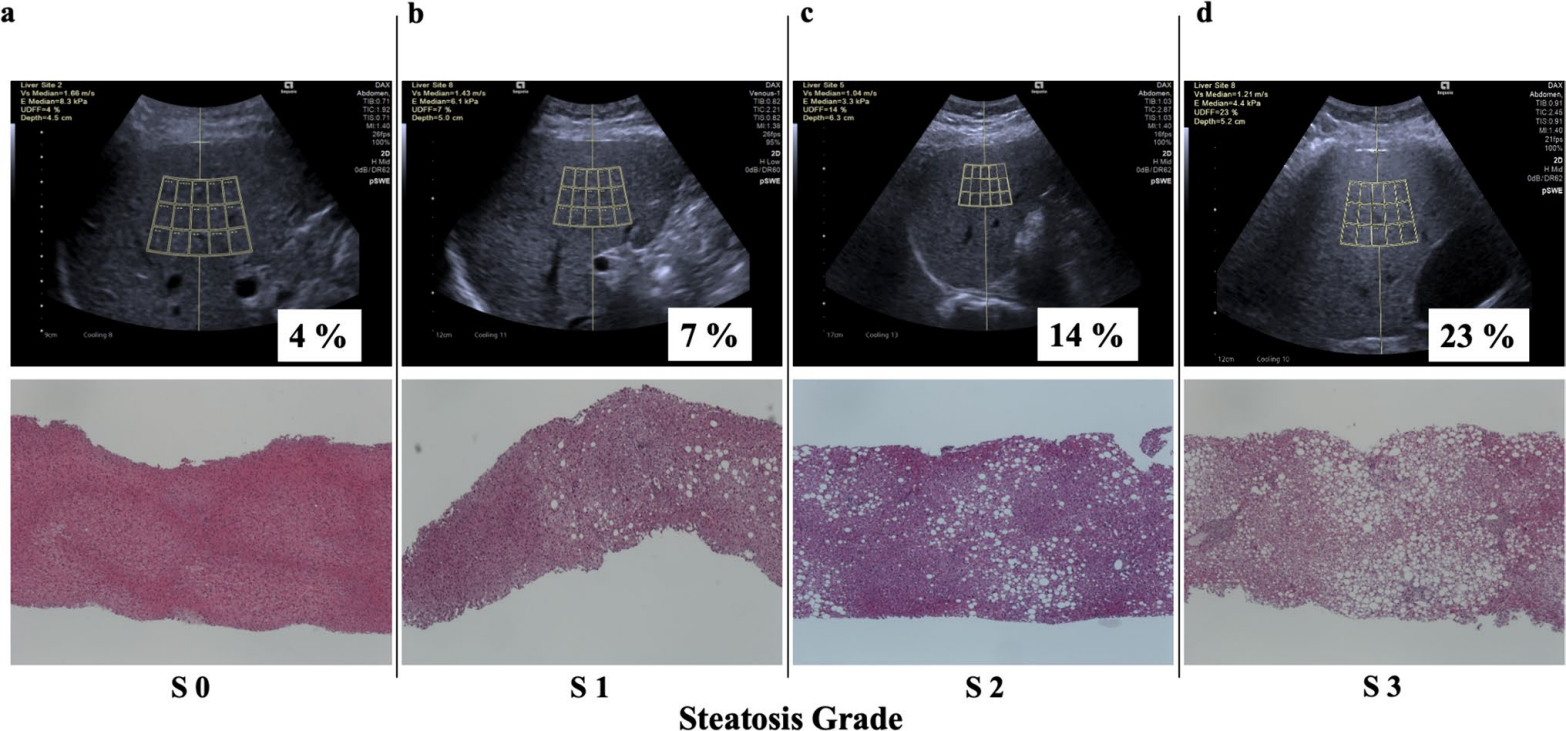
Representative cases. a Steatosis grade 0 with 4% of UDFF measurement. b Steatosis grade 1 with 7% of UDFF measurement. c Steatosis grade 2 with 14% of UDFF measurement. d Steatosis grade 3 with a UDFF value of 23%.  *UDFF* ultrasound-derived fat fraction
